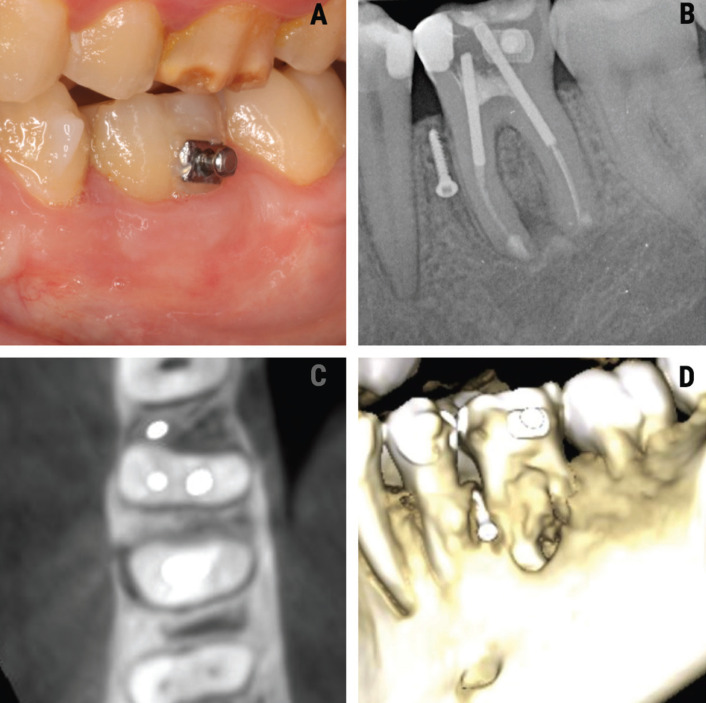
A. Intraoral view of tooth 3.6 after one year. B. Periapical radiograph showing bone trabeculation between the roots of the lower left first molar. C. Tomographic view showing the formation of bone around the furrow of 3.6. one year after periapical surgery. D. Three-dimensional tomographic (CBCT) reconstruction of the current condition of tooth 3.6.
